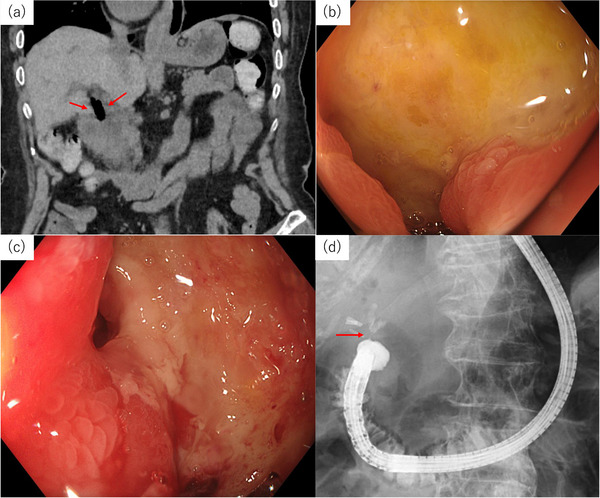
(a) Non‐contrast CT demonstrated the presence of air within the common bile duct (red arrows). (b, c) Endoscopic image showing an ulcerative lesion with a small fistula in the duodenal bulb. (d) Under fluoroscopic guidance, the endoscope was applied to the fistula, and a contrast study demonstrated leakage of a contrast medium into the common bile duct (red arrow). CT, computed tomography.
